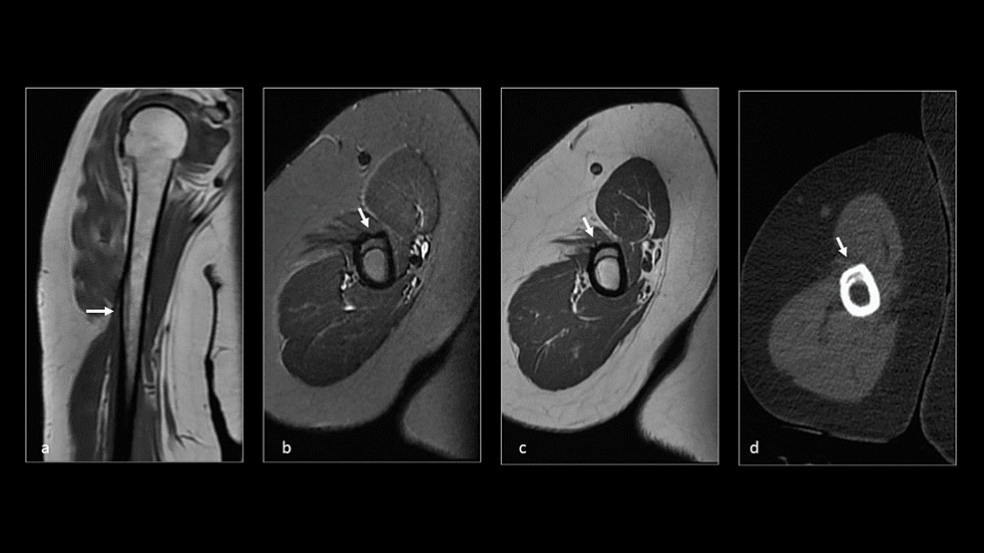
CT and MRI evaluation of the right shoulder. (a) Coronal T1WI shows an isointense area of cortical thickening. (b and c) Axial T2 STIR and T1WI hyperintense area of cortical thickening compared to the normal cortical bone signal intensity at the region of the deltoid insertion in the right humerus with no evidence of adjacent marrow and soft tissue edema. (d) Axial CT shows well-defined cortical thickening, expansion, and mild irregularity on the endosteal surface with irregular intracortical lucency at the deltoid muscle attachment area. CT, computed tomography; MRI, magnetic resonance imaging; T1WI, T1-weighted image; T2 STIR, T2-weighted short-tau inversion recovery
